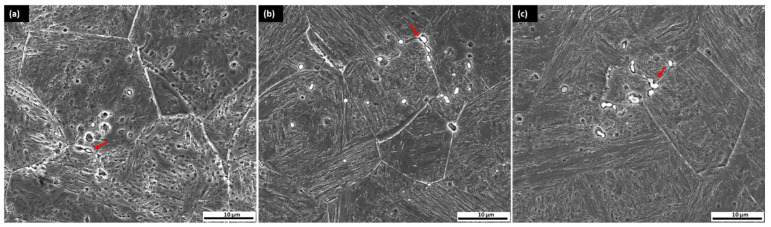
FESEM microstructure of sample: (a) with cryogenic treatment CT/T2; (b) CT2/T5; (c) CT2/T10.
